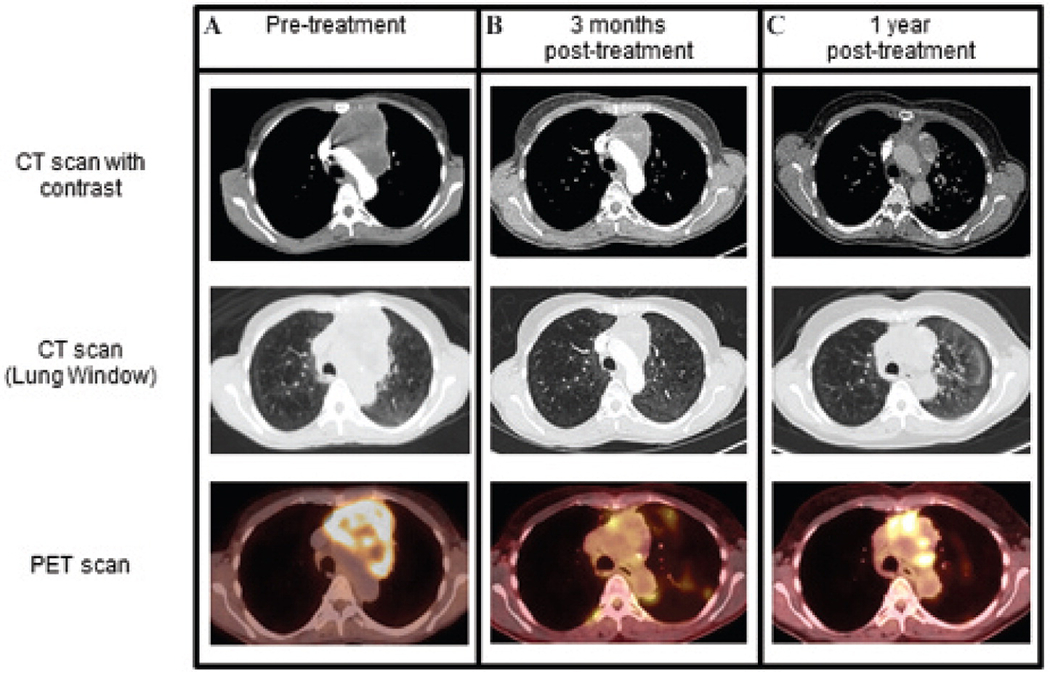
Imaging presentation of an anterior mediastinal mass in patient D.H. *A*, On initial imaging, the patient presented with an anterior mediastinal mass with heterogeneous enhancement that measured 8.6 × 7.8 cm in size (upper CT image) (maximum SUV 10.7, lower [^18^F]FDG PET image). The great vessels were displaced medially from the left side, and the upper lobe branch of the left pulmonary artery was displaced inferolaterally. *B*, After chemoradiation, surveillance imaging demonstrated a reduction in the mass to 5.6 × 3.1 cm (upper CT image), with only a small rim of increased tracer uptake along the right medial and inferior margins and a maximal SUV of 6.1 (lower fusion [^18^F]FDG PET/CT image). Given the favorable response, further therapy was deferred. *C*, One year later, there was an increase in the [^18^F]FDG uptake in the medial and inferior aspects of the anterior mediastinal mass (SUV maximum 8.7, lower fusion [^18^F]FDG PET/CT image), suspicious for tumor recurrence.
